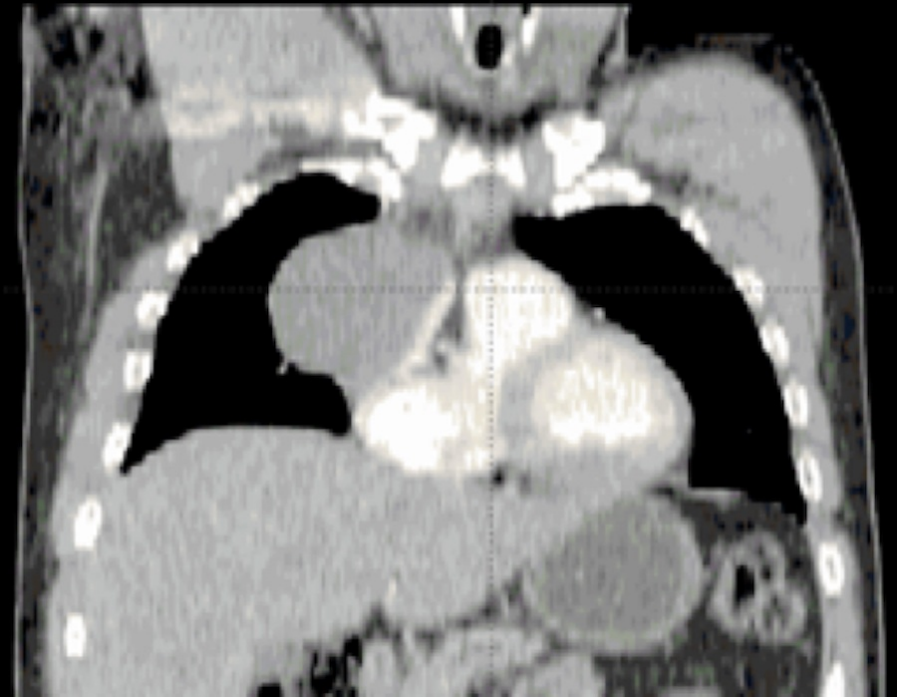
Coronal contrast-enhanced computed tomography scan of thorax showing the right anterior mediastinal mass.
